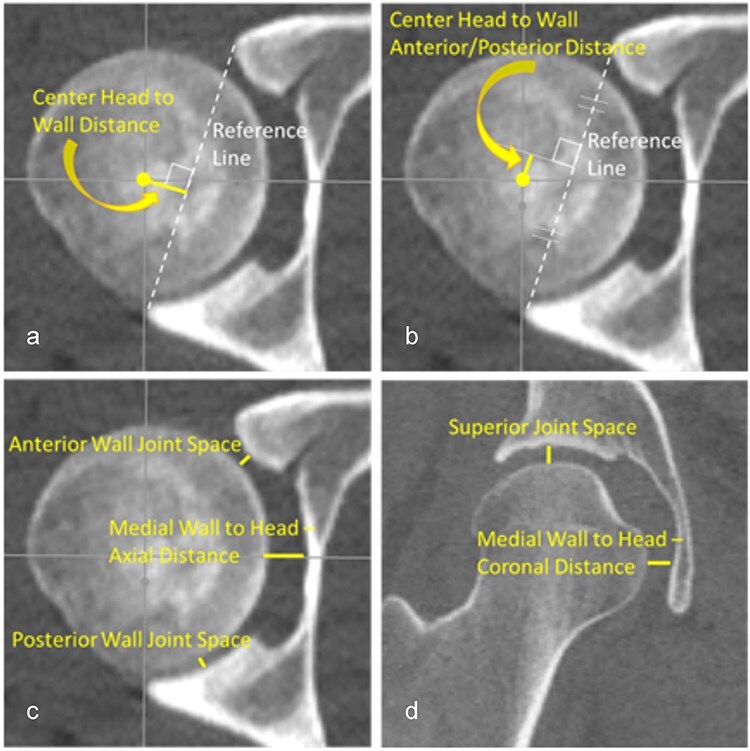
A total of seven 2D manual measurements were performed on each CT scan: (a) the distance from the FHC to a line connecting the anterior and posterior rim of the acetabulum, (b) the distance from the FHC to the perpendicular bisector of the rim-to-rim line, (c) the anterior and posterior wall joint space along with the medial wall to head distance in the axial plane, and (d) the superior joint space and the medial wall to head distance in the coronal plane. All measurements were taken on a single axial and a coronal plane image that was verified in three orthogonal imaging planes to pass through the center of the left and right femoral heads.
